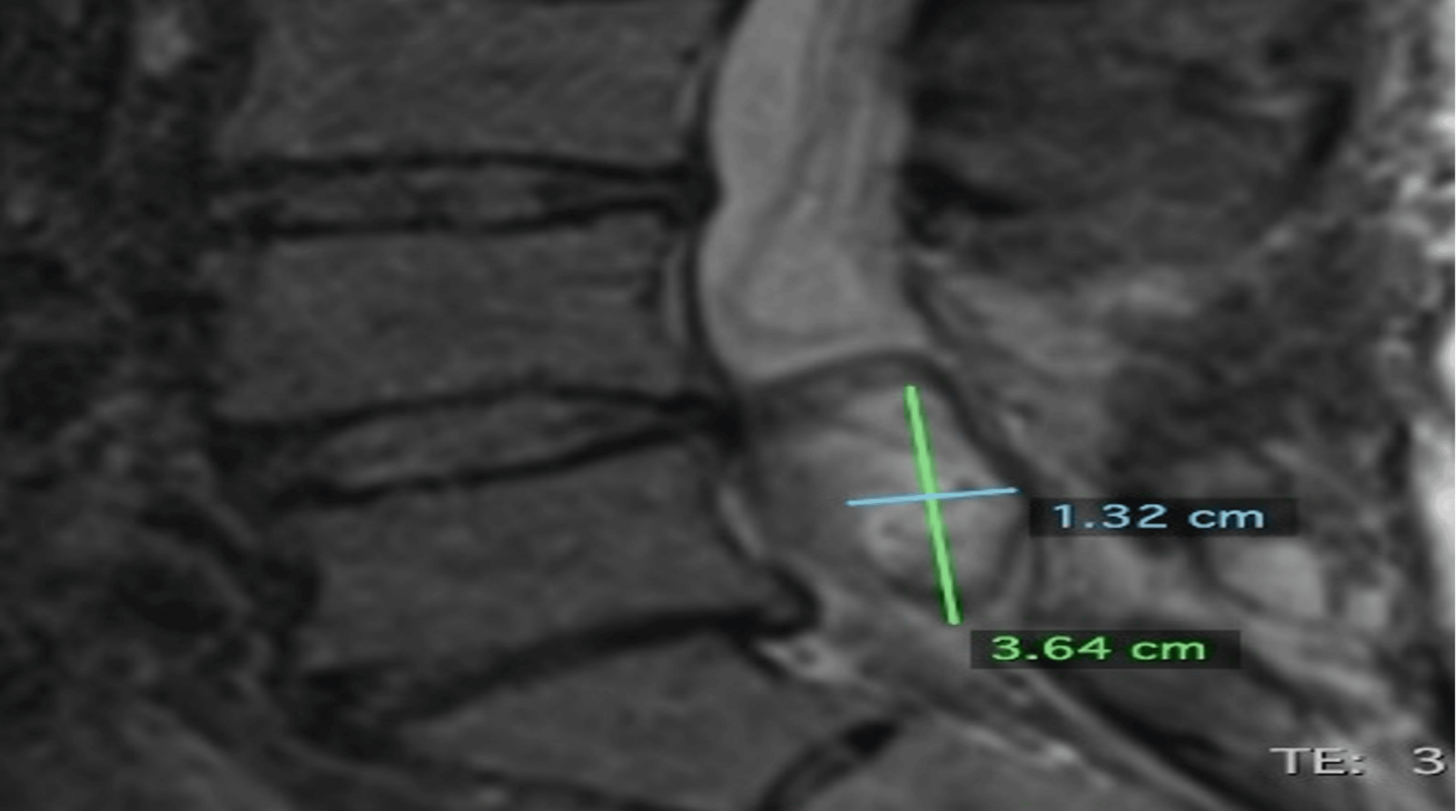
Pre-cryoablation sagittal image from an MRI dated May 3, 2023. The tumor measured 3.64 cm superiorly to inferiorly and 1.32 cm anteriorly to posteriorly.
